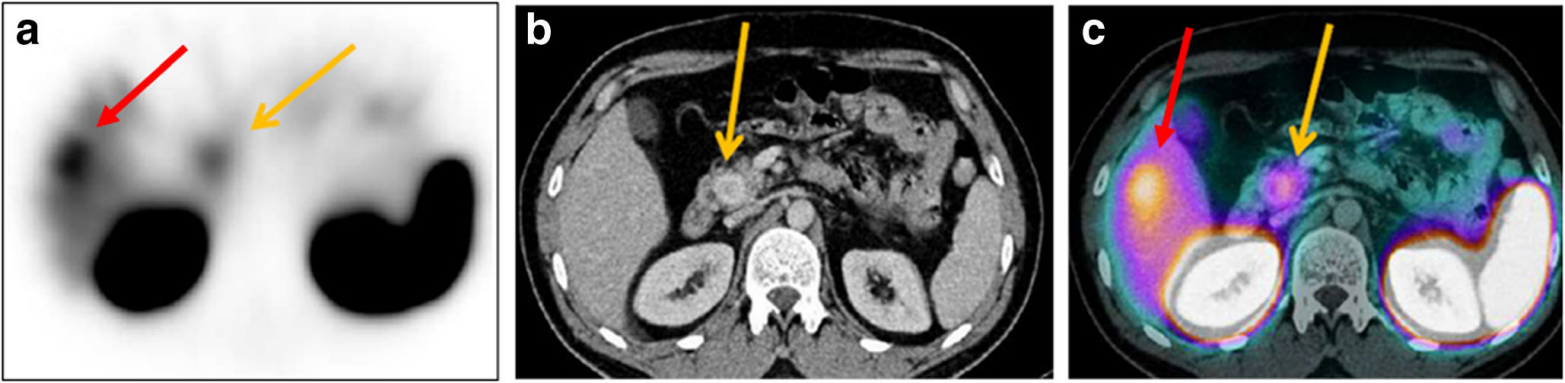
^111^In-somatostatin SPECT/CT in neuroendocrine neoplasms. A 34-year-old patient with biochemical suspicion of NEN and a lesion in the head of pancreas on CT was referred for ^111^In-somatostatin scintigraphy for staging. SPECT (a) shows two foci of intense tracer uptake in the upper abdomen, localized by contrast-enhanced CT (b) to a hypervascular primary lesion in the head of the pancreas and an isodense metastasis in the right lobe of the liver (arrows), confirmed on SPECT/CT (c)
